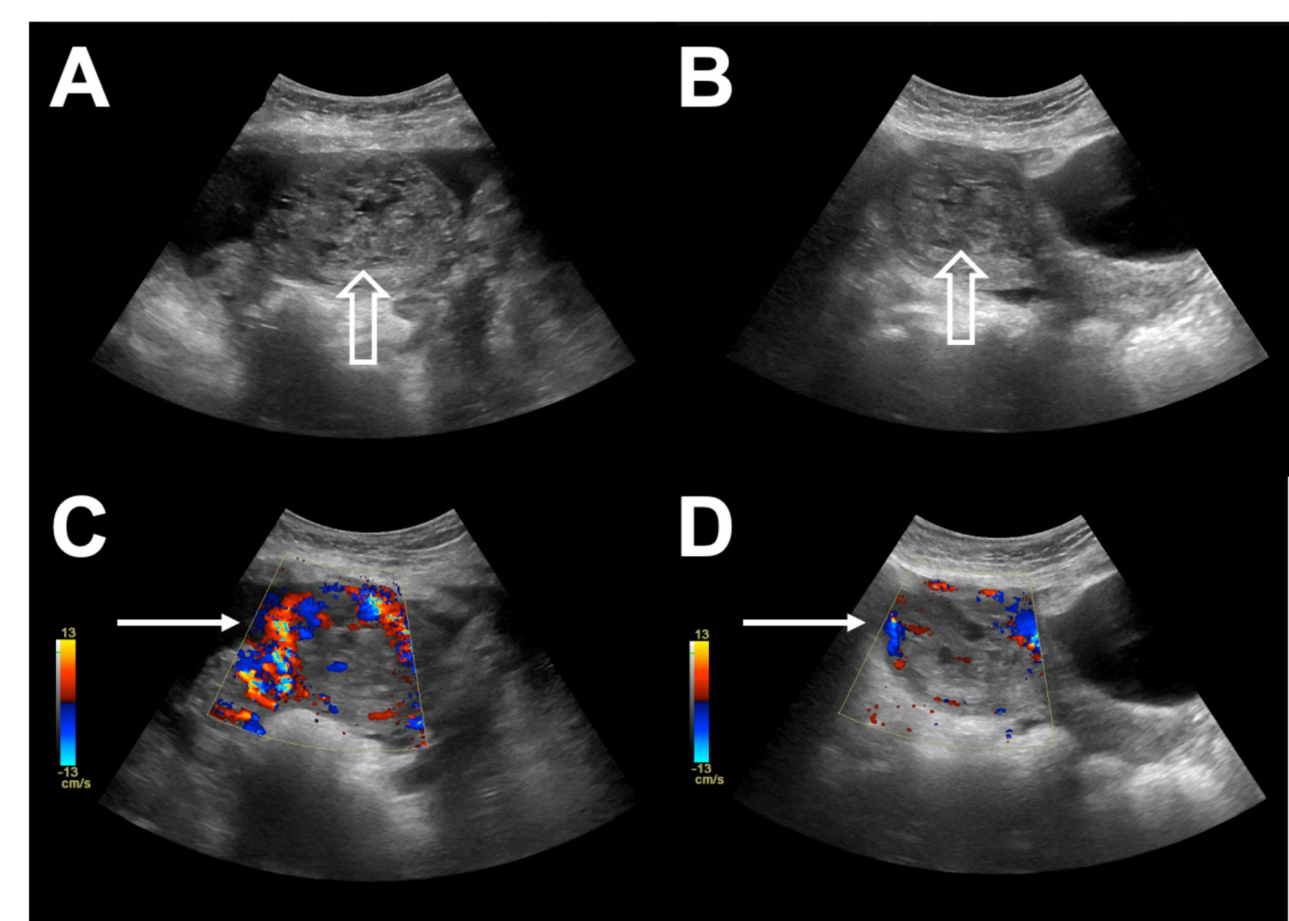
A and B. Initial transabdominal ultrasound, Transverse view (A and C) and sagittal view (B and D) revealing a hypervascular uterus (white arrows) with internal echogenic material (blank arrows).
